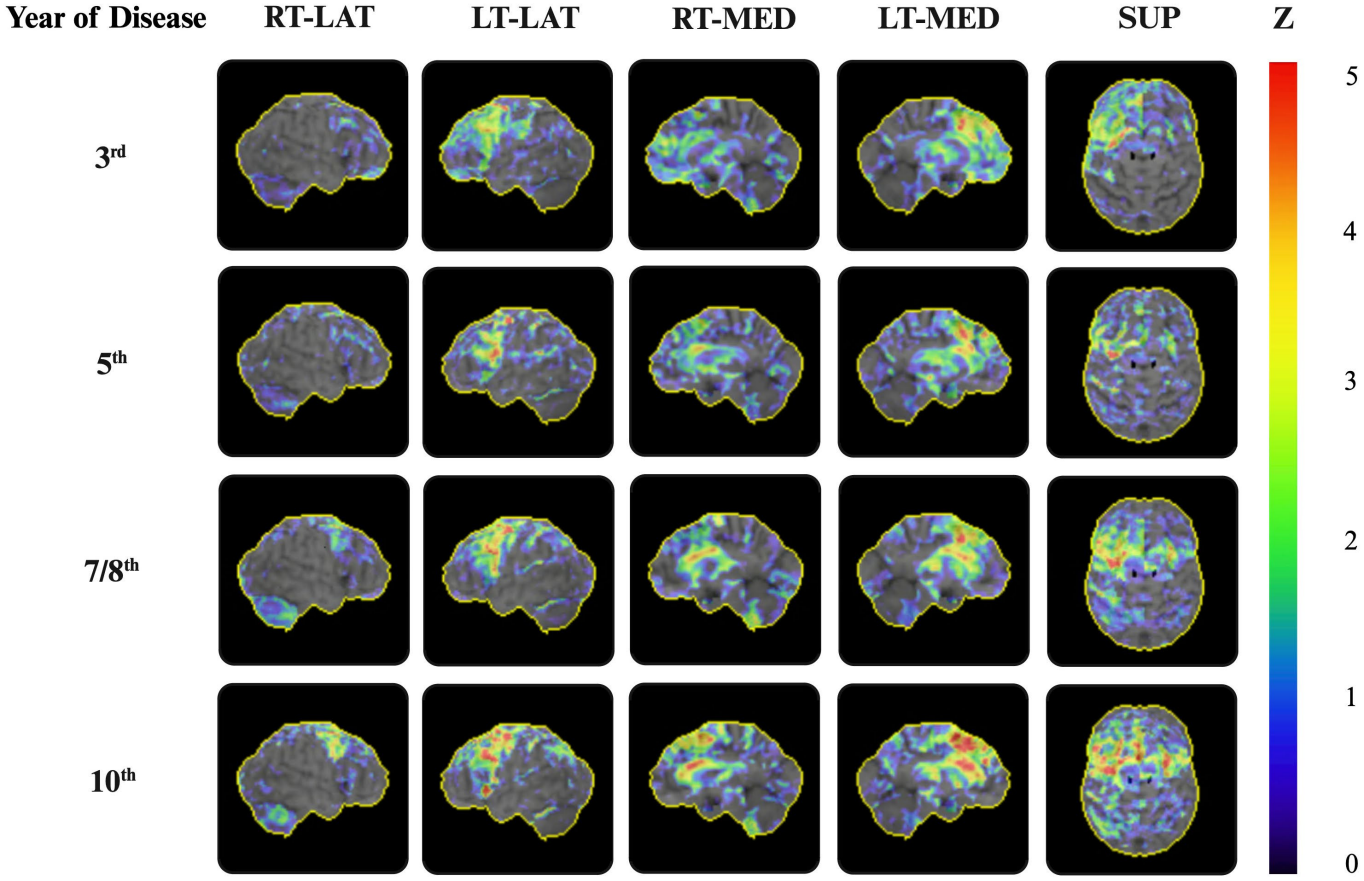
2-[^18^F]FDG-PET during the disease progression. Representative 3D surface projections of the 2-[^18^F]FDG-PET on the sagittal and axial planes, using the NEUROSTAT software, show significant frontal hypometabolism with accentuation on the left side erased during the disease progression. From the eighth year of the disease progression onward, the reduced metabolic uptake became more pronounced, predominantly in the left-sided premotor and prefrontal cortices, as well as in the dorsolateral area and basal ganglia. 3D surface projections depict the regional distribution of Z-transformed deviations in the glucose uptake in the patient, relative to a reference database, visualized using color-coded *z*-scores. Z = *z*-score. RT-LAT, right-lateral; LT-LAT, left-lateral; RT-MED, right-medial; LT-MED, left-medial; SUP, superior.
